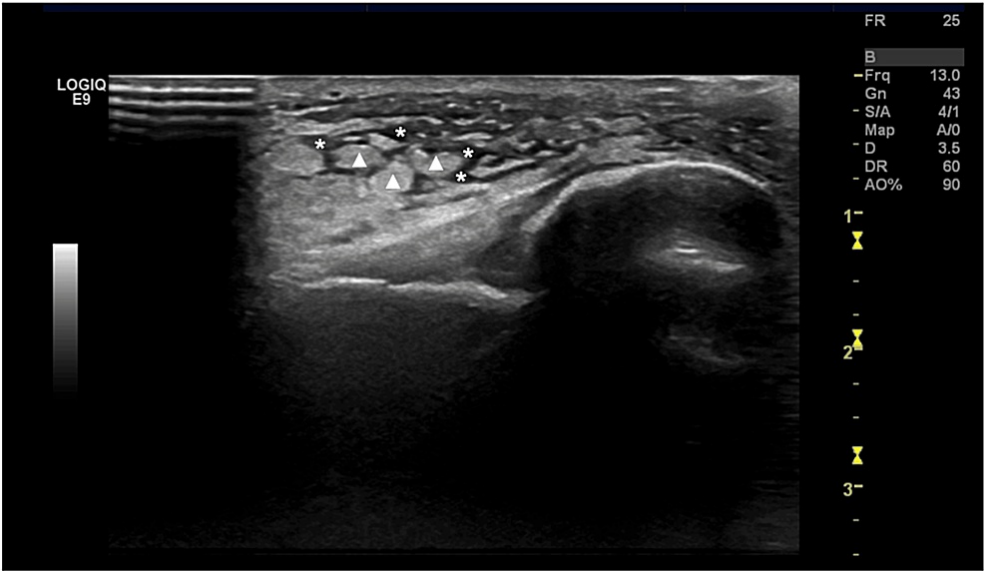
Grayscale ultrasound showing hypoechoic subcutaneous tissue (asterisks), decreased echogenicity of the dermis, and increased size and echogenicity of the fatty lobules (triangles) of the subcutaneous tissue.
